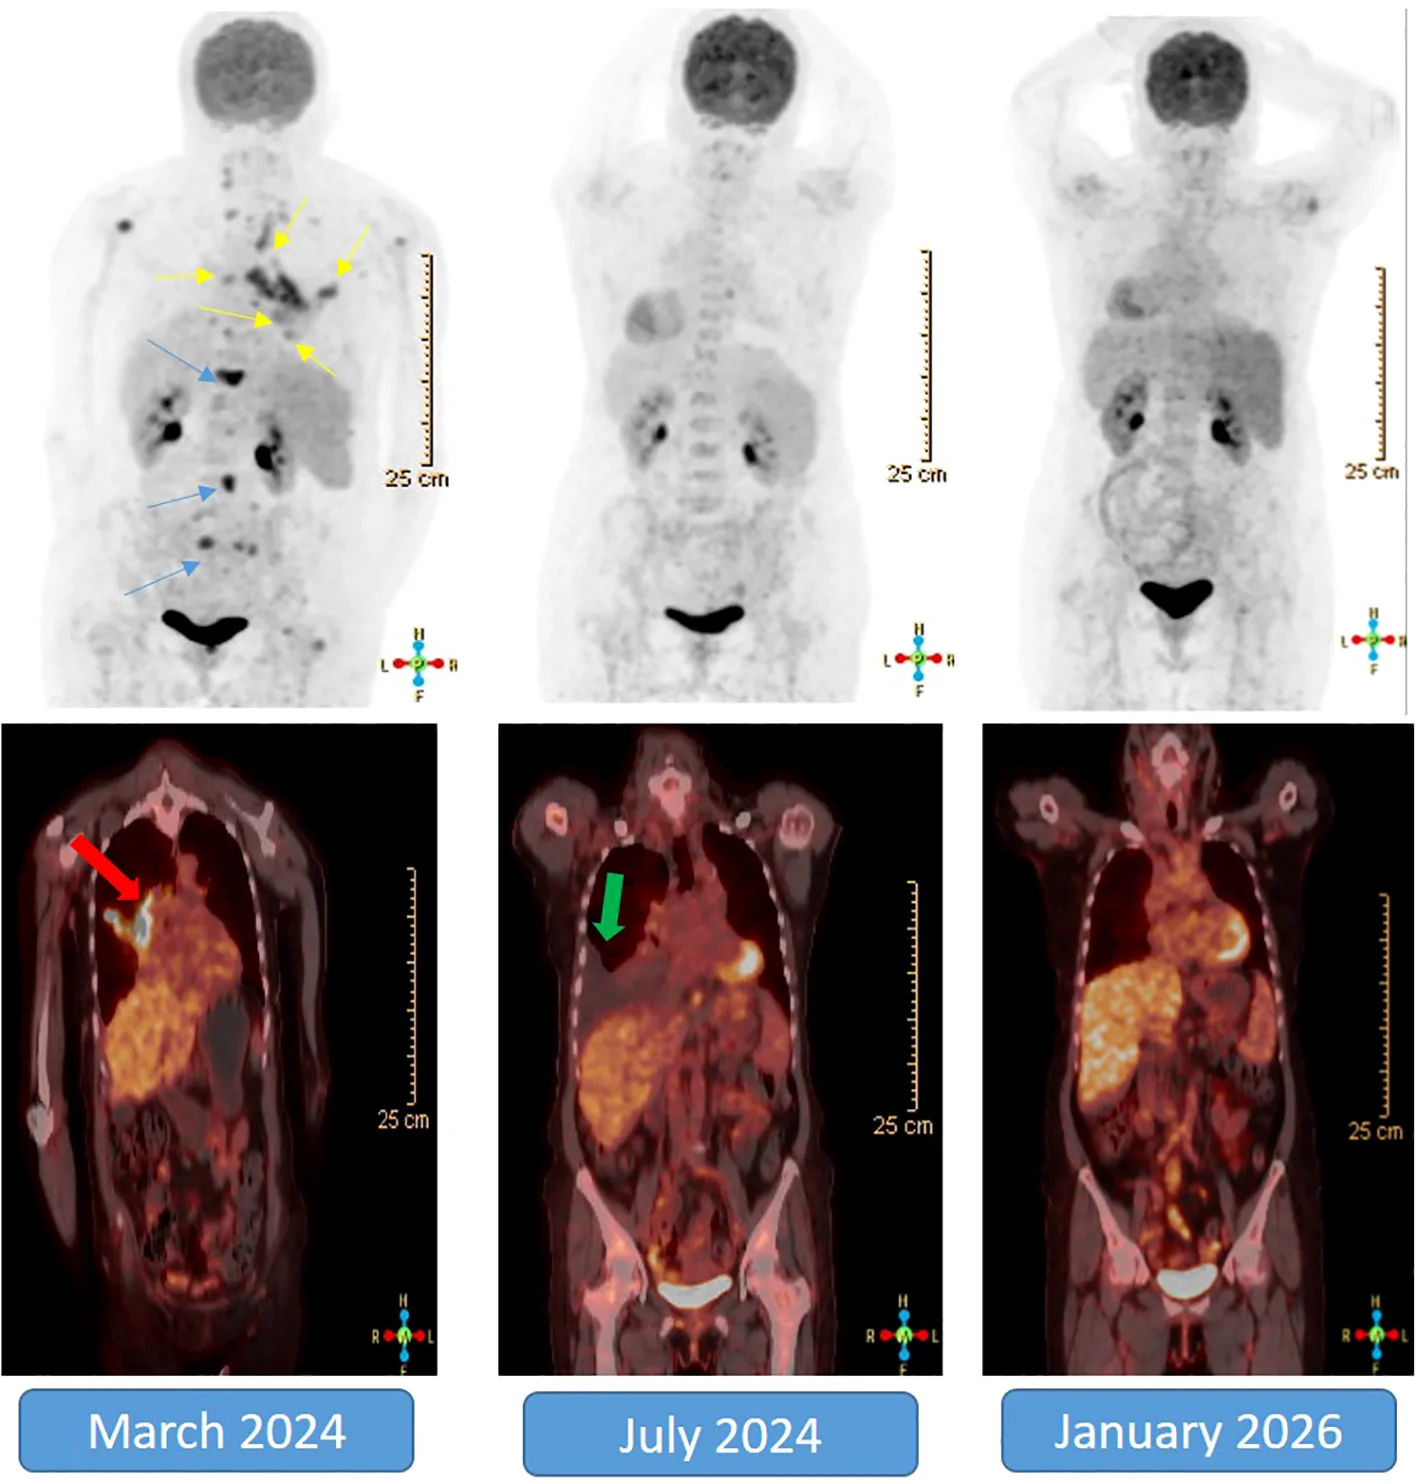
Response to treatment on FDG-PET scan. The red arrow points to the primary FDG-avid malignant lesion in the right middle lobe, with the avidity resolving by July 2024 and remaining resolved in January 2026. The green arrow points to the right pleural effusion present at diagnosis, which had resolved by the January 2026 scan. Blue arrows point to the lytic bone lesions seen at diagnosis that had resolved on follow-up scans. Yellow arrows point to the FDG-avid hilar and mediastinal lymph nodes seen at diagnosis that had resolved on follow-up scans.
